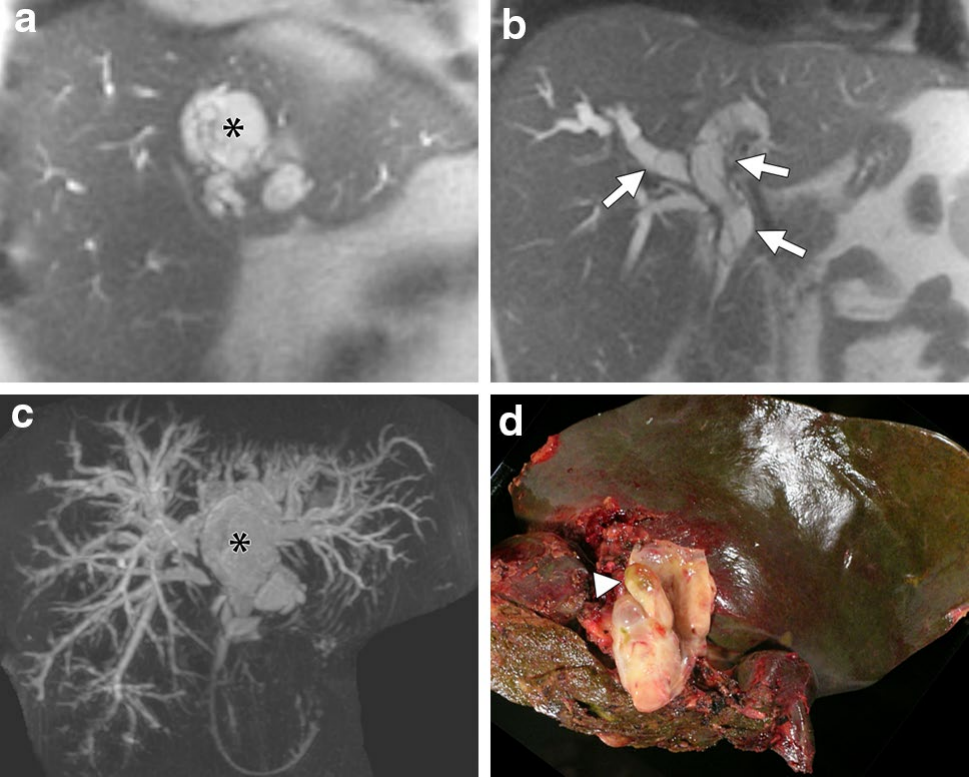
A 27-year-old woman, who presented with abdominal pain and jaundice, had a 63 mm multilocular cystic mass in segment IV of the left lobe of the liver (a, single shot fast spin echo coronal image. asterisk). Multilocular cystic mass also could be seen in both hepatic ducts extending to the extrahepatic duct, which indicated intraductal tumor prolapse (b, single shot fast spin echo coronal image. arrows). Peripheral bile duct dilation was also seen in MRCP (c, 2D-MRCP). The gross pathology picture demonstrates the biliary prolapse of the mucinous cystic neoplasm of the liver (d, gross pathology picture. arrowhead). Only MRI was available to review. Both readers could tell the biliary prolapse
